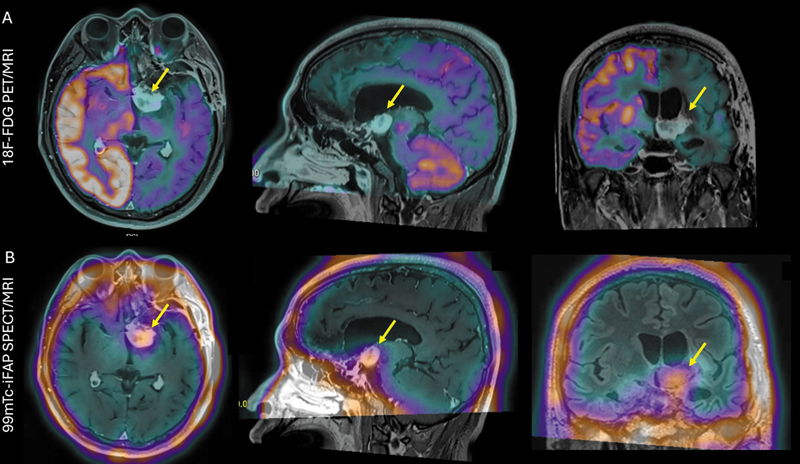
Axial, sagittal, and coronal projection images obtained through fusion of magnetic resonance imaging (MRI) and molecular imaging: (
